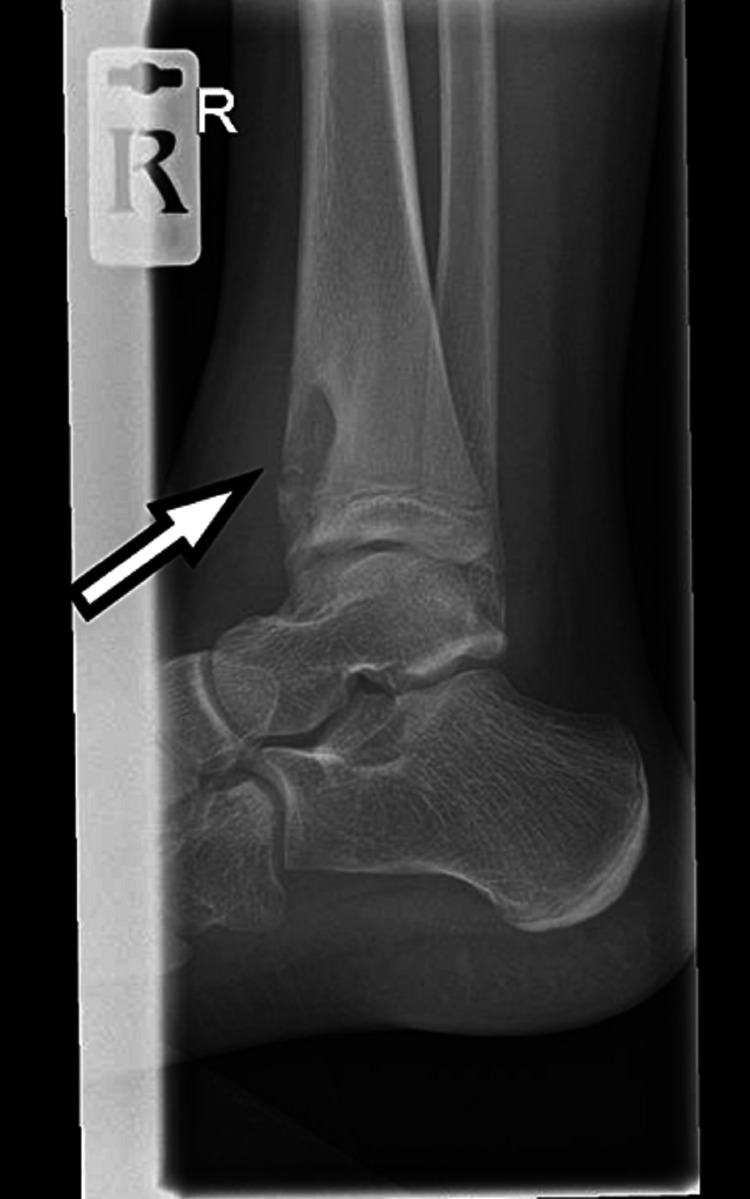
Lateral radiograph showing the lesion in the distal tibia involving the epiphysis, physis, and metaphysis
